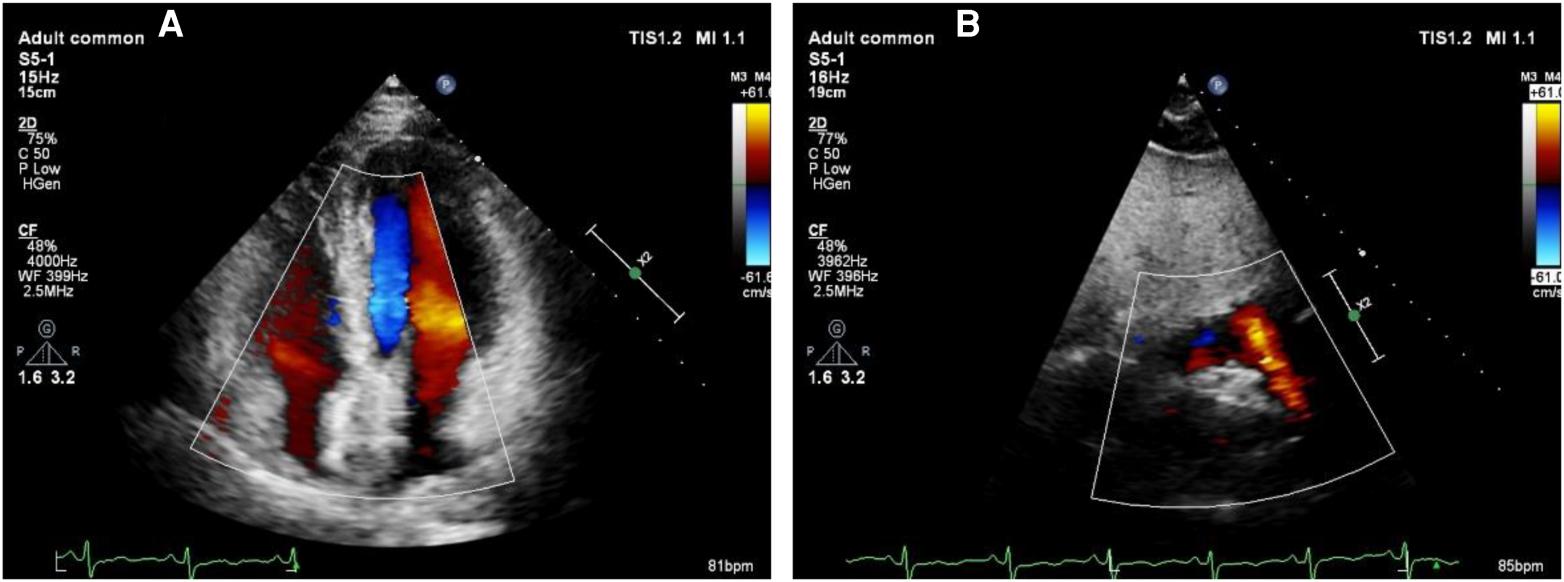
Echocardiography to exclude pericardial tamponade. (A) The left ventricular ejection fraction of 65% with no evidence of pericardial tamponade was found. (B) Strong echo of the occluder can be seen in atrial septum.
